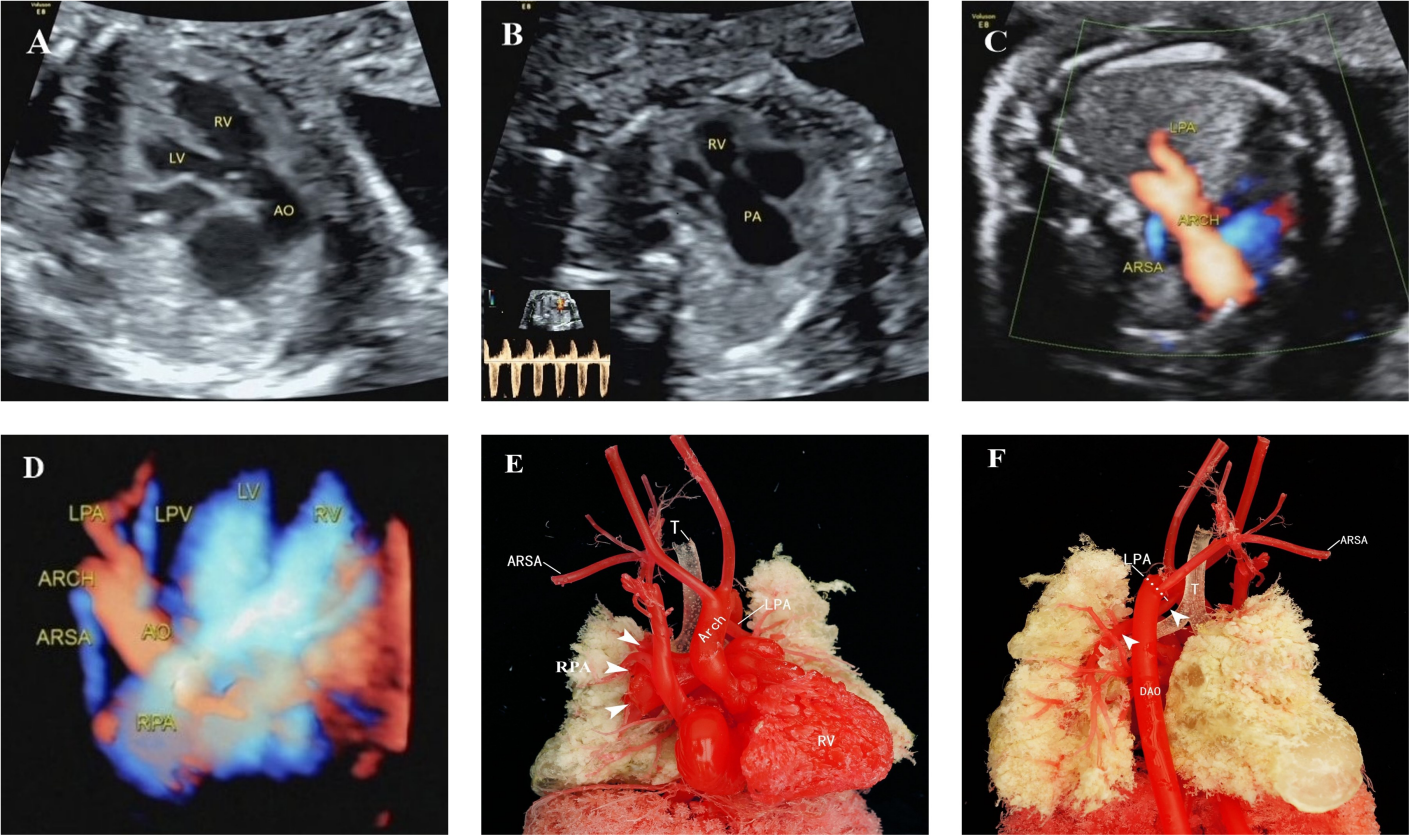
Unilateral absence of pulmonary artery with absent pulmonary valve in tetralogy of Fallot. (A) Outflow tract section showed the ventricular septal defect and aortic riding span. (B) Indistinct pulmonary valves, small development of the pulmonary annulus, significantly widened pulmonary artery and high-speed blood flow signal of two-way round trip in the spectrum Doppler. (C,D) Right subclavian artery was vagus, and the left pulmonary artery originated from the transverse arch of the aorta. (E,F) Anatomical casting showed that the absence of the pulmonary valve created a “whale’s tail” sign in the right pulmonary artery and that the left pulmonary artery originated from the transverse arch of the aorta. LV, left ventricle; RV, right ventricle; ARSA, aberrant right subclavian artery; LPV, left pulmonary vein; ARCH, aortic arch; DAO, descending aorta.
